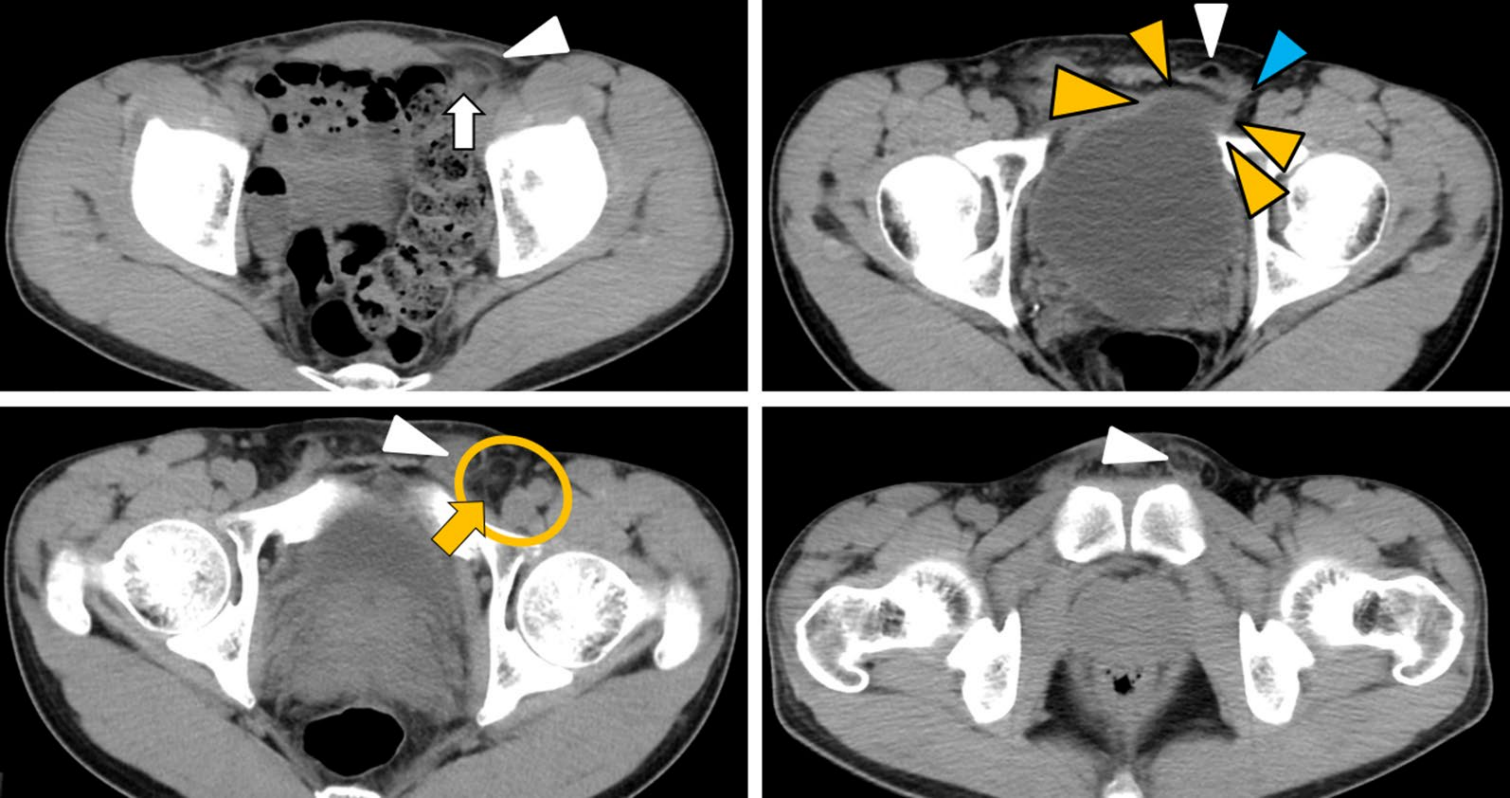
Computed tomography (CT) performed five years before admission. CT showed a left indirect inguinal hernia (white arrowheads) which protruded from the outside of the inferior epigastric vessels (white arrow). A portion of the bladder (yellow arrowheads) was pulled to the left femoral ring (blue arrowhead). The small adipose tissue (yellow arrow) was observed in the left femoral canal (yellow circle)
